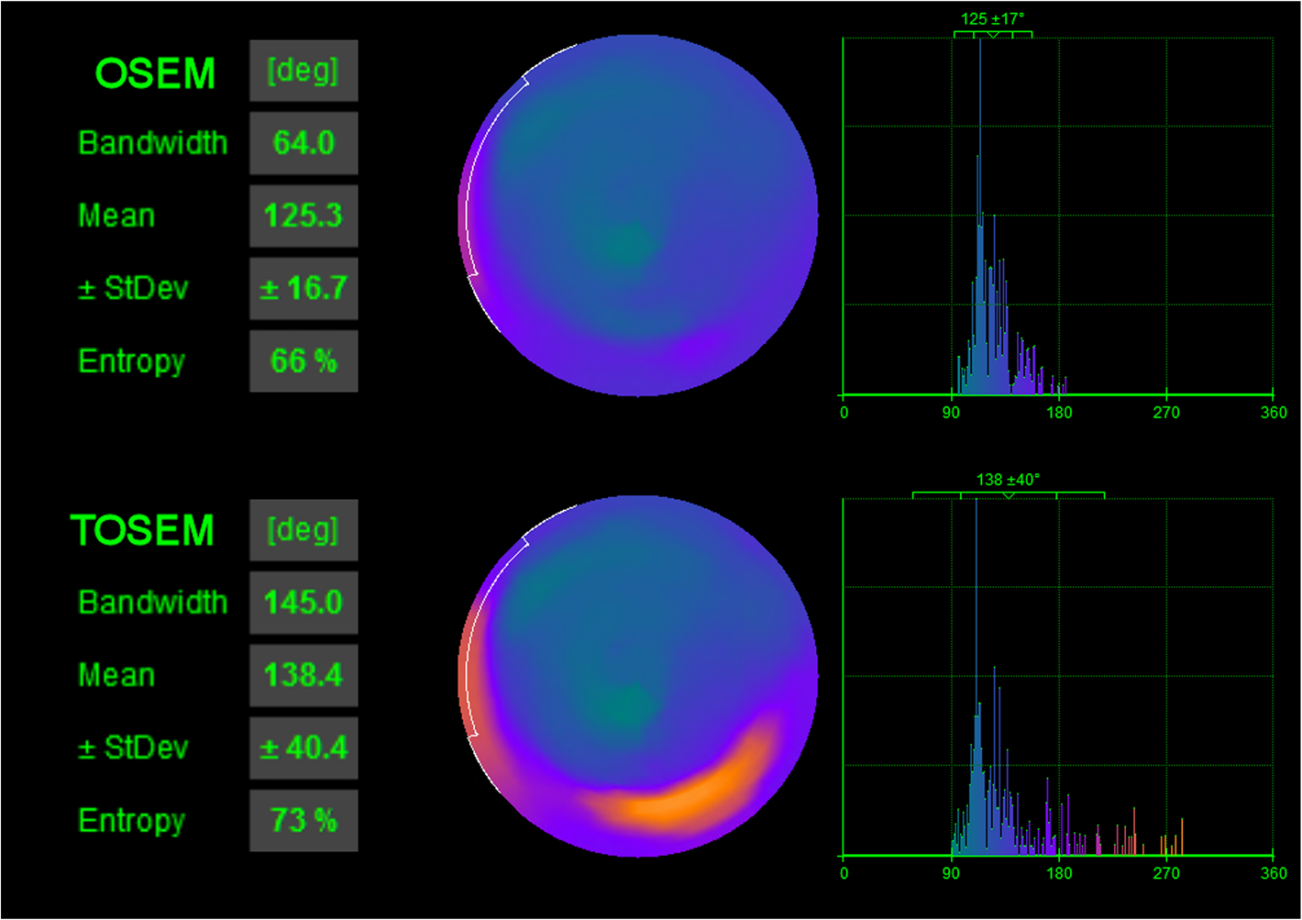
Phase analysis results from Quantitative Gated SPECT 2012 program for the same patient as in Figure 1. Phase analysis assessed from OSEM-reconstructed images yielded mildly abnormal results, with the polar map showing relatively well-synchronized function. On the contrary, phase analysis assessed from TOSEM-reconstructed images yielded considerably abnormal results, with the polar map clearly showing delayed contraction in the inferior basal region of the left ventricle. For this patient, the calculated activity ratio value was 73%
